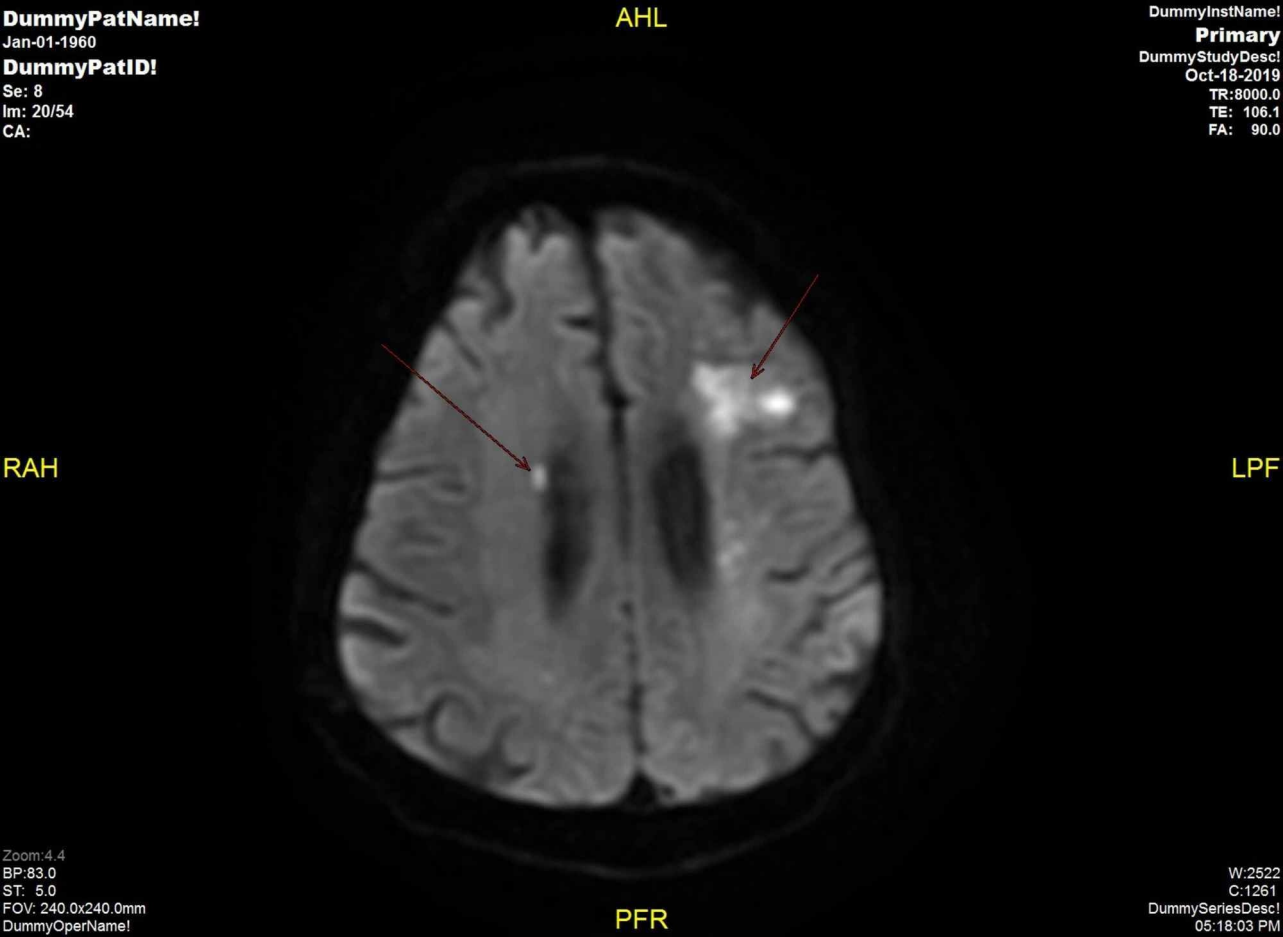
MRI showing embolic stroke
